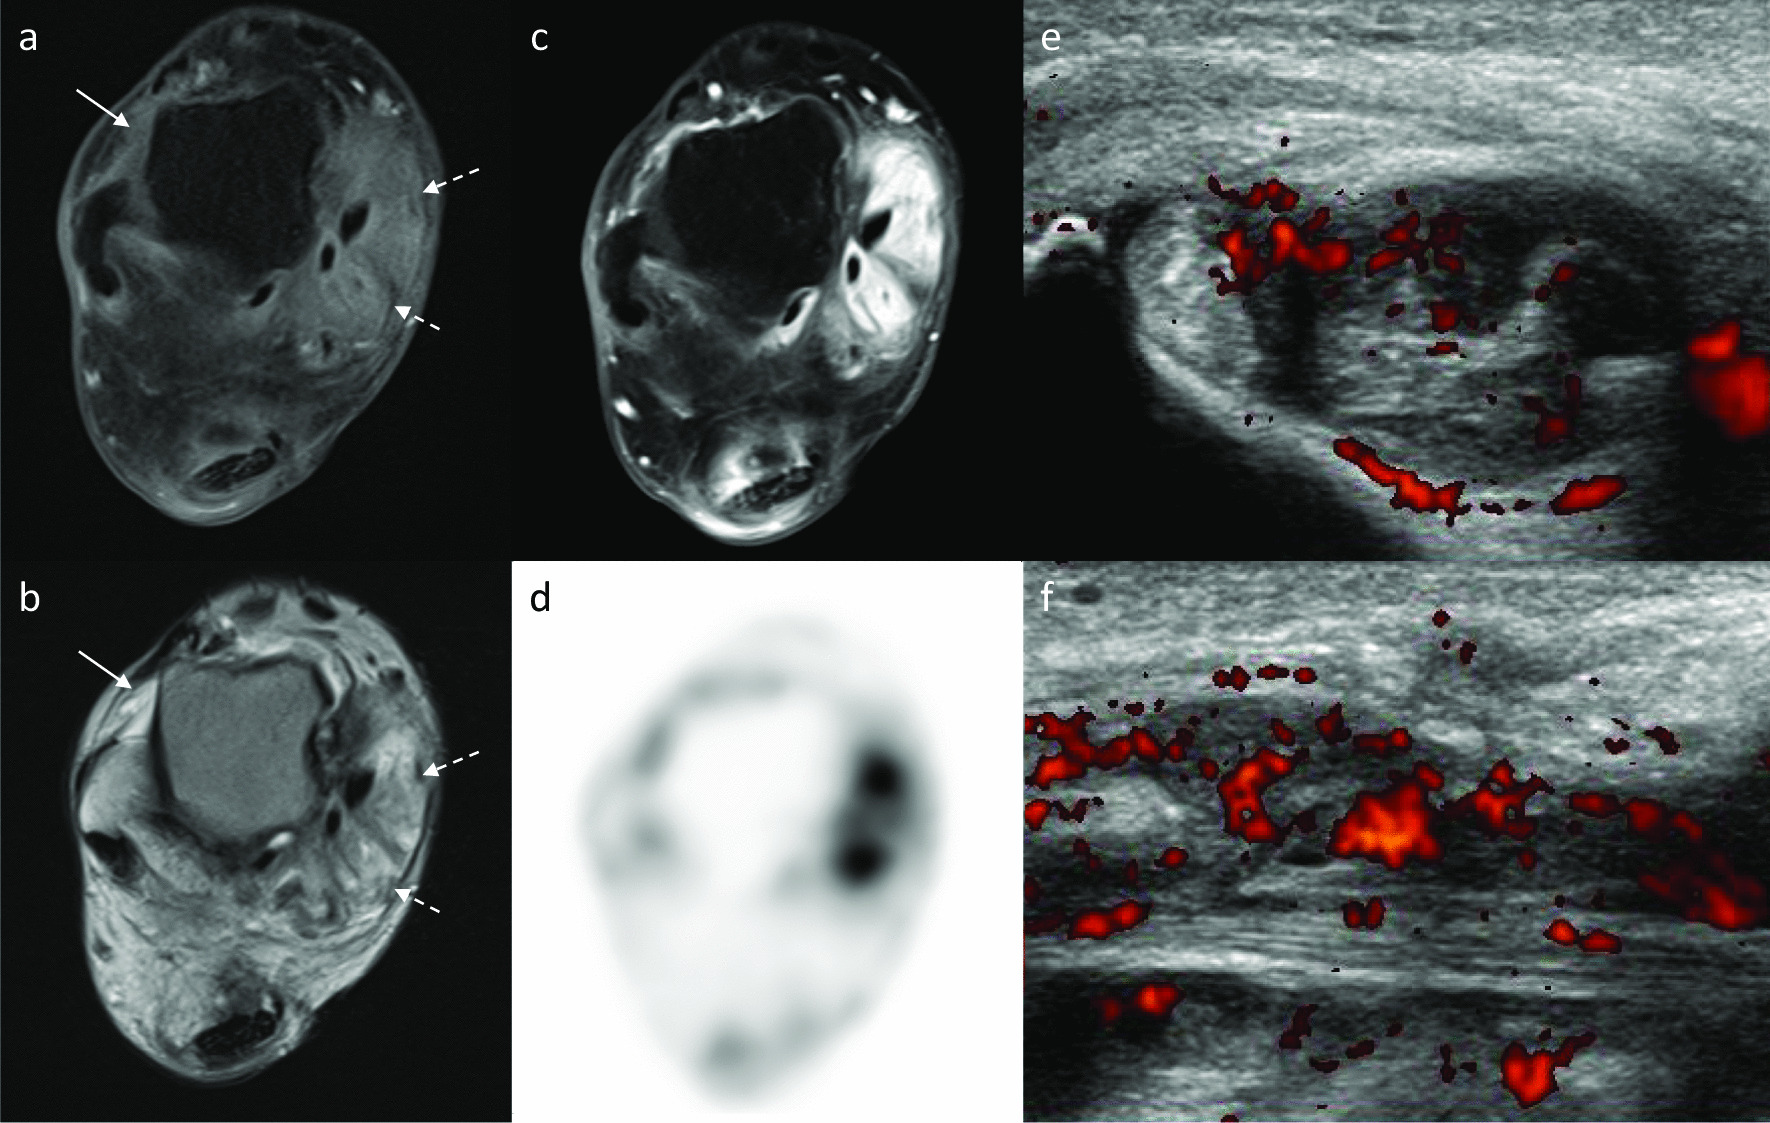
Images of right talocrural joint level in a 42-year-old woman with peripheral spondyloarthritis. a Axial T1-weighted and b axial T2-weighted images show synovial thickening anterior to talus (arrows) and around the medial flexor tendon (dashed arrows). These are enhanced in the fat-saturated contrast enhanced T1-weighted image (c), and FDG uptake is corresponding to synovial thickening (d). Color Doppler ultrasonographic images at the right medial flexor tendon (e; short axis, f; long axis) also show tenosynovitis as a hypoechoic area with heterogenous hyperemia
